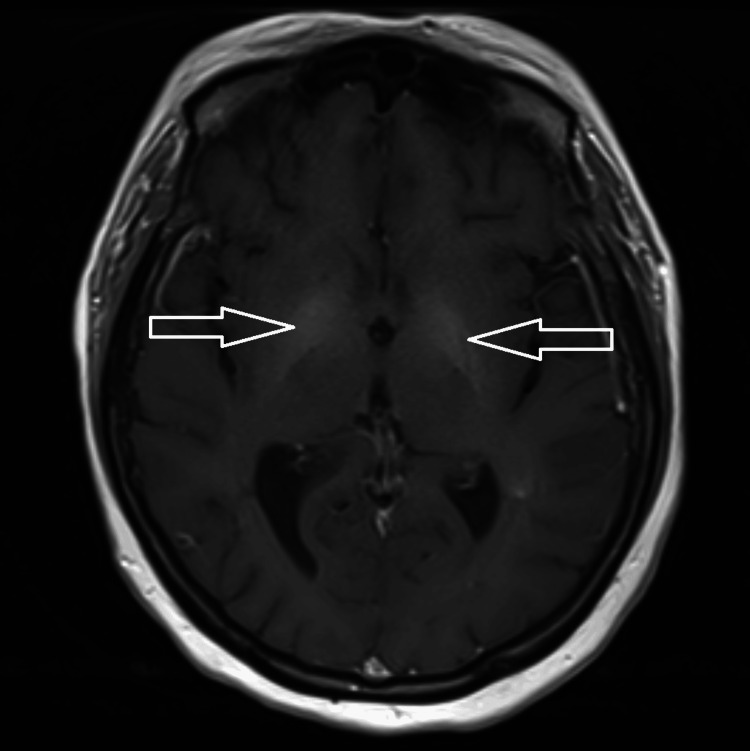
Axial T1-weighted MRI of the brain, with the arrows pointing to the globus pallidus hyperintensity bilaterally
